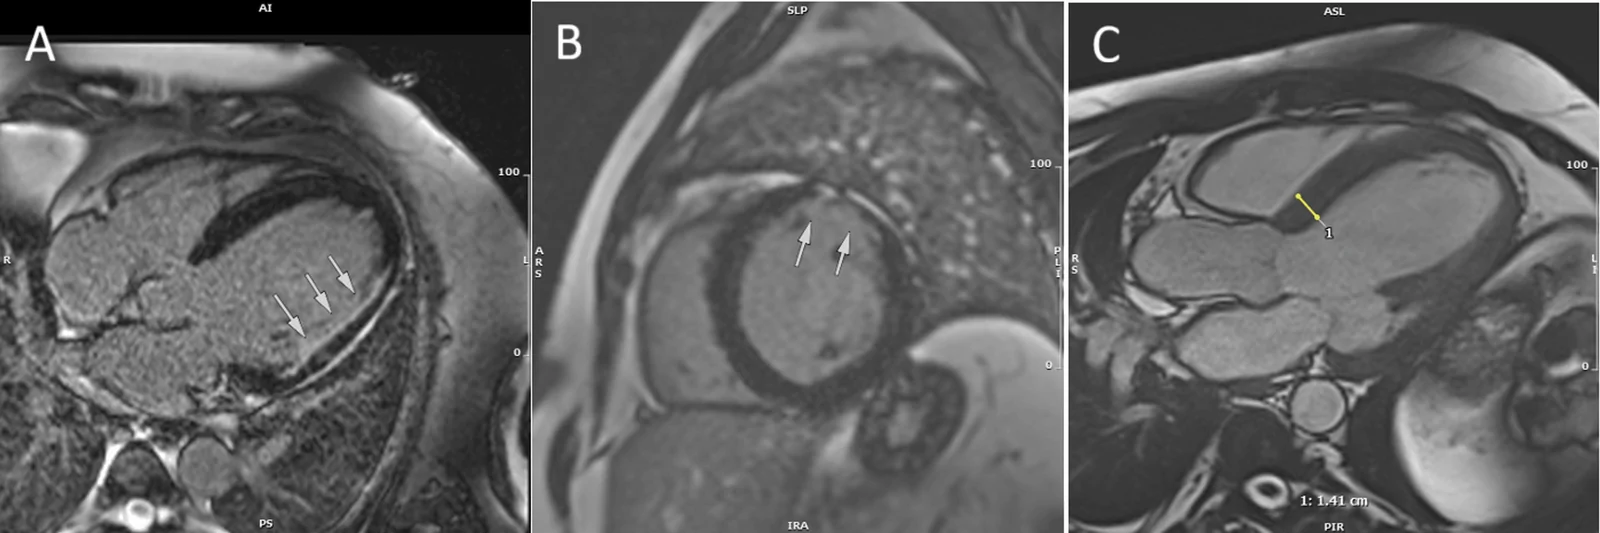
Cardiac MRI findings. (A) Post-gadolinium four-chamber view demonstrating a small area of subendocardial infarction in the anterolateral wall (arrows). (B) Post-gadolinium short-axis view confirming the same area of subendocardial ischemic injury (arrows). (C) Three-chamber view in diastole, showing mild concentric left ventricular hypertrophy (upper limit of left ventricular wall thickness is 12mm).
